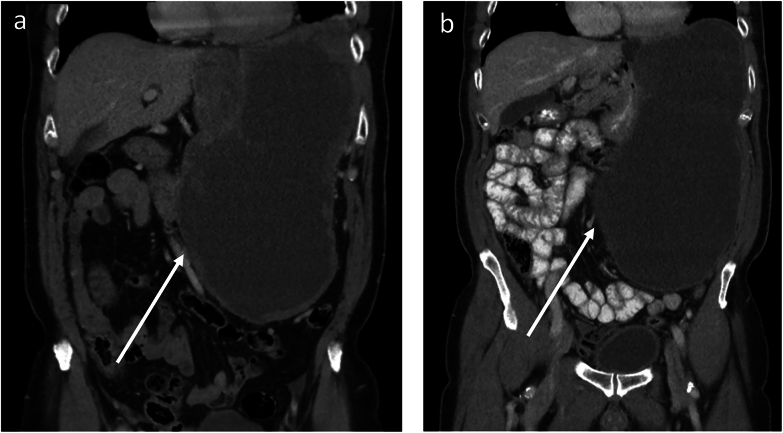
Coronal portal venous phase CE-CT images of a patient diagnosed with a large high-risk gastric GIST (white arrows). a) On baseline CE-CT imaging, the tumoural site of origin was difficult to assess and the tumour was adherent to the left hemidiaphragm. b) The preoperative CE-CT image. After 5.2 months of TKI treatment (imatinib), minimal tumour shrinkage was observed, and the visualisation of the tumour attachment was not improved. During the surgical procedure, the diaphragm was partially opened because of the tumour adherence to the left hemidiaphragm. During the process of tumour detachment, the tumour ruptured, causing potential iatrogenic tumour exposure to the dissection field. This patient did not benefit from TKI treatment, as there was no de-escalation in surgical strategy and surgical complexity was not reduced.
